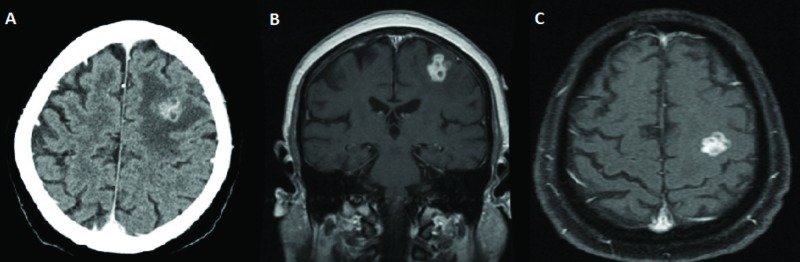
Preoperative imaging demonstrating a multi-lobulated, relatively uniformly contrast-enhancing lesion in the left frontal lobe just anterior to the motor cortex. A) Axial CT with contrast; B) Coronal T1 MRI with contrast; C) Axial T1 MRI with and without contrast CT: computed tomography; MRI: magnetic resonance imaging
